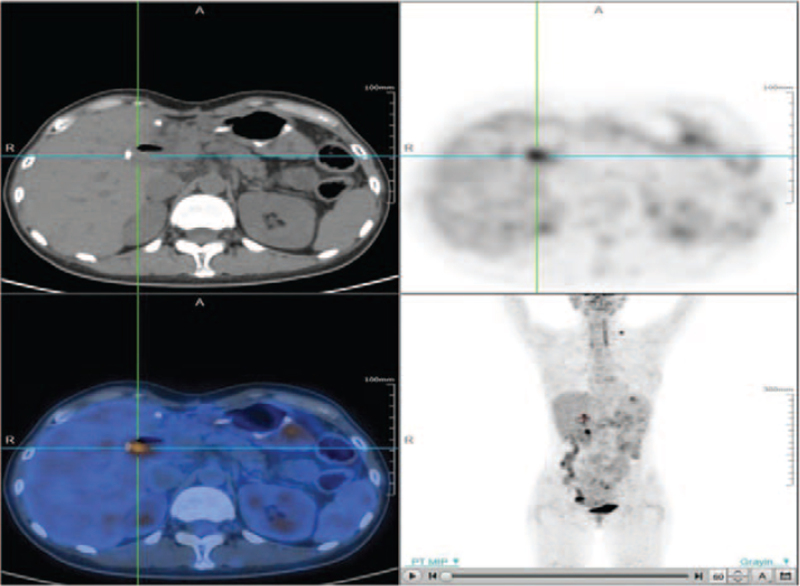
After 6 cycles of immunotherapy combined with chemotherapy, PET/CT showed that the tumor was significantly reduced.
